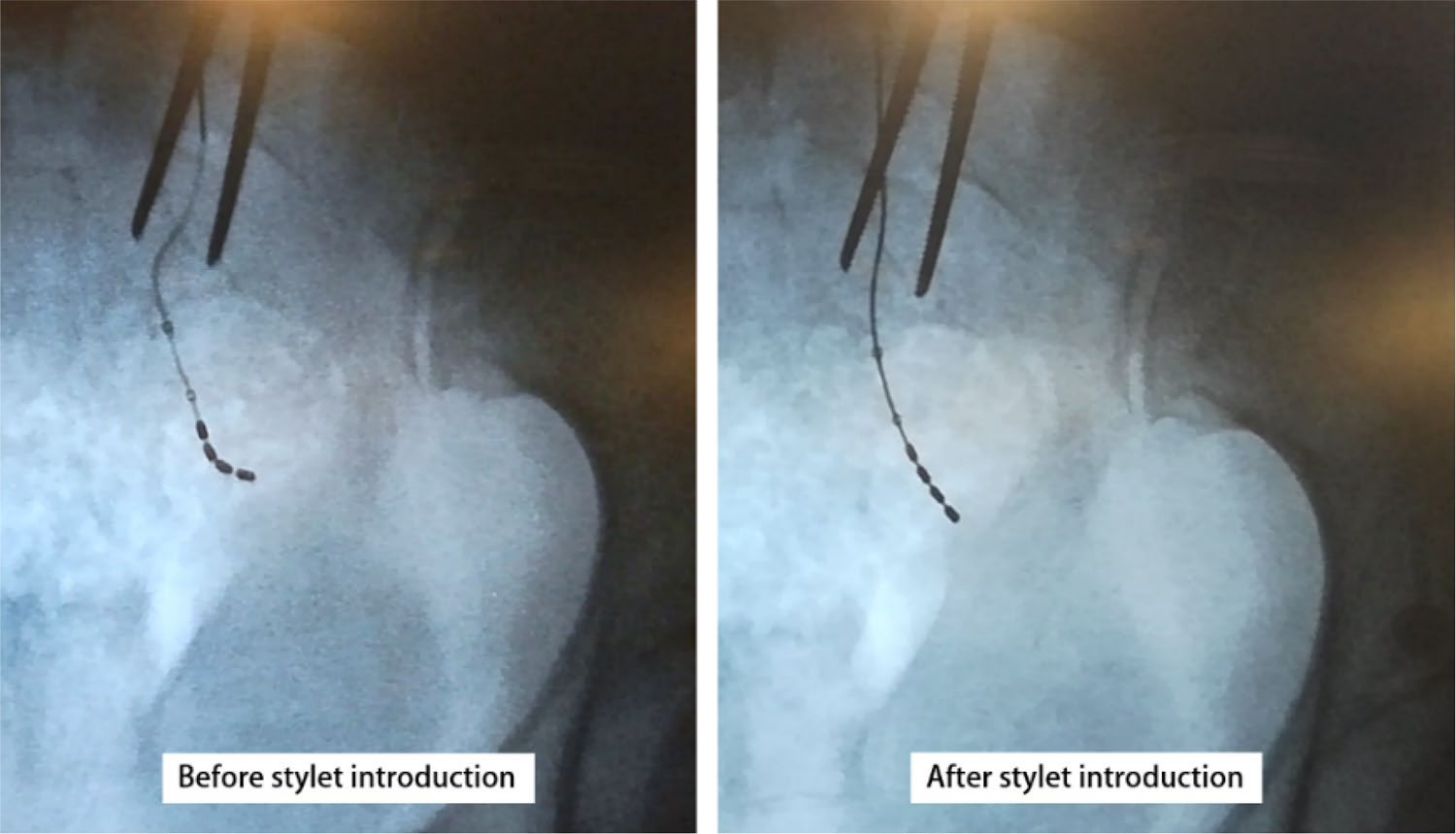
X-ray imaging during the lead removal procedure. It is possible to see the effect of using the stylet in straightening the electrode. The use of X-ray imaging is not part of the procedure. It was performed in the first patients to verify the manoeuvre and is here reported for a clinical purpose
